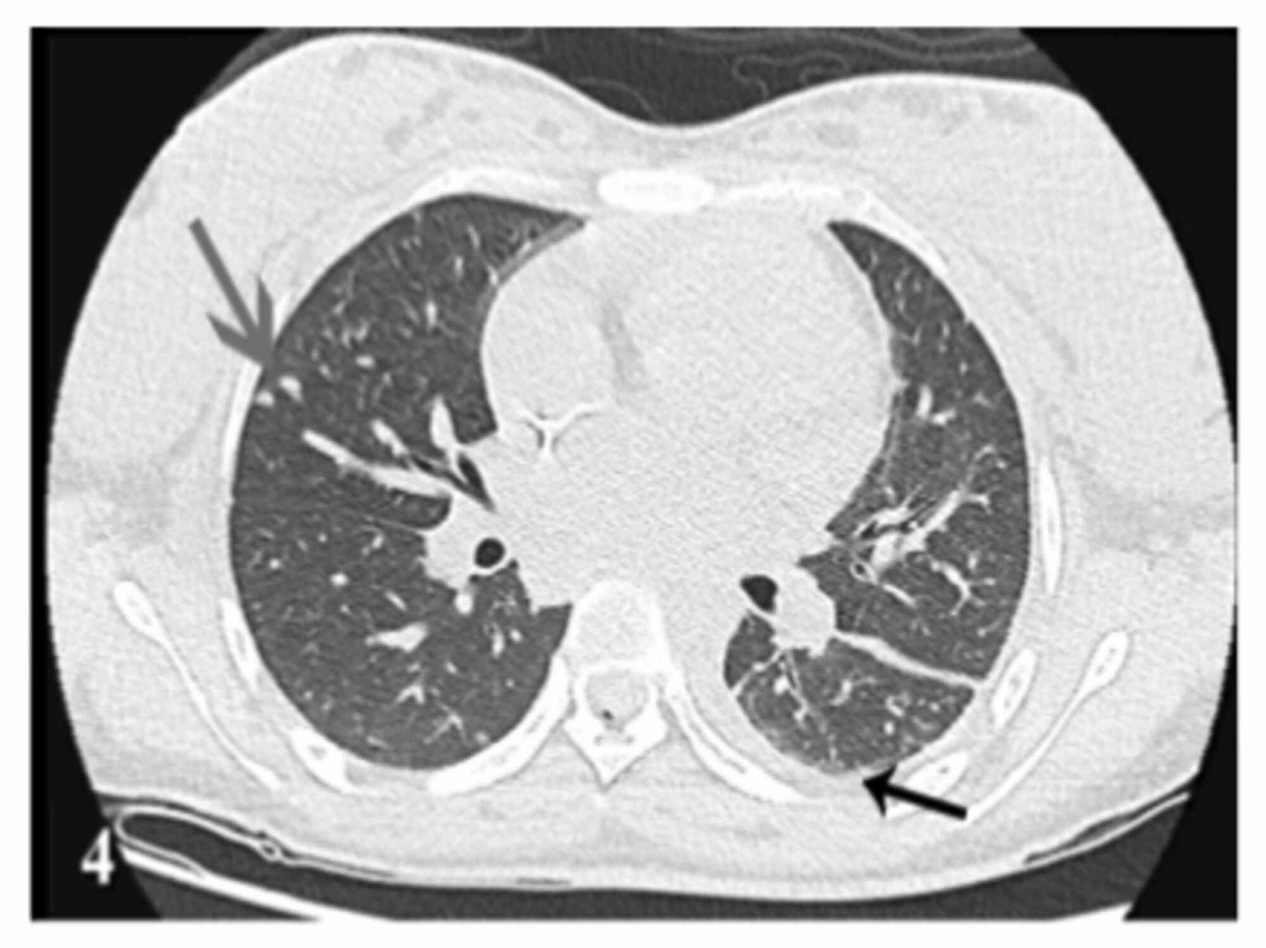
Follow-up CT chest without contrast Follow-up CT chest without contrast after two weeks showing improving pneumonia (black arrow). Also, many solid noncalcified nodules decreased in size (gray arrow).
